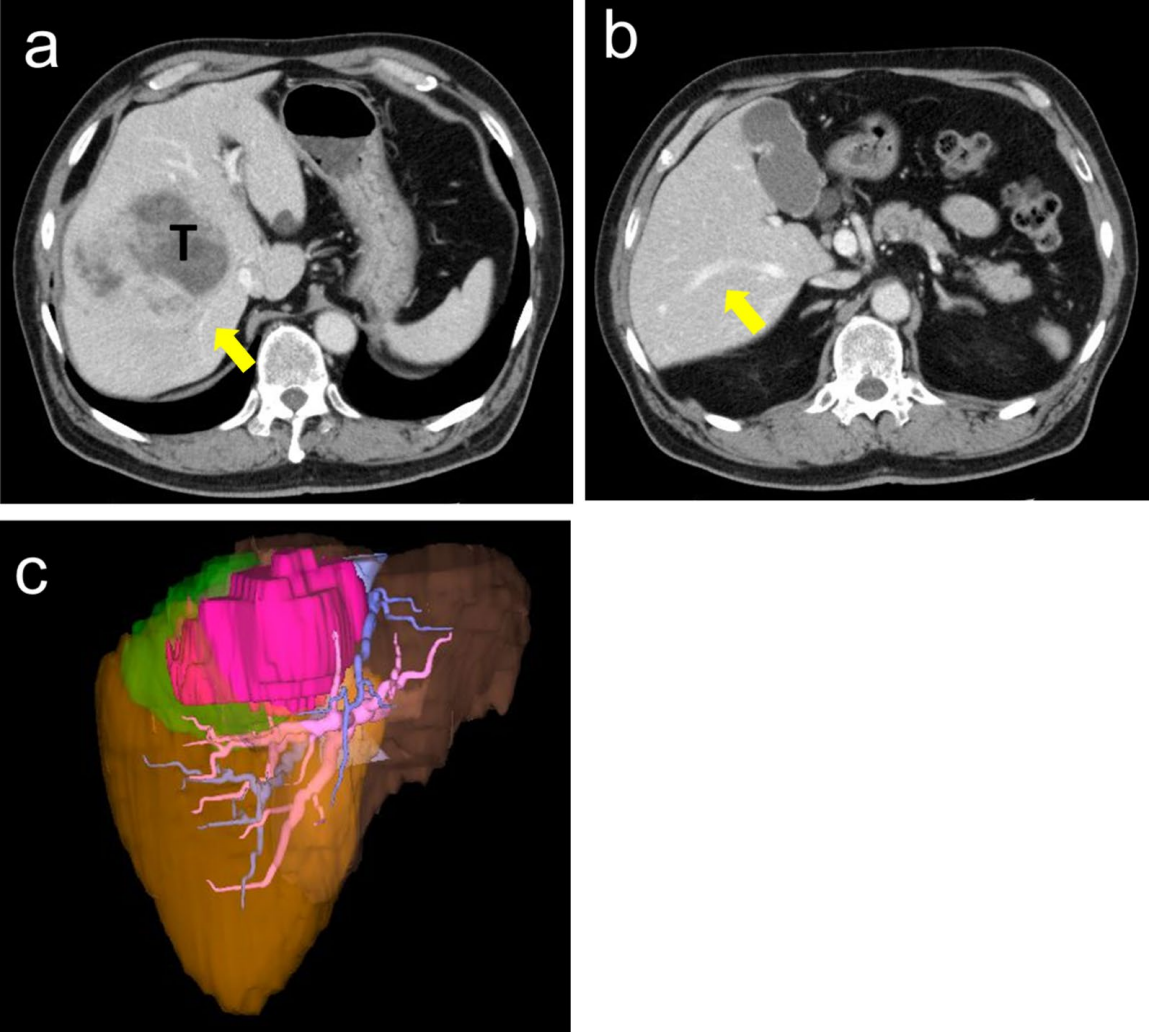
Hepatocellular carcinoma occupying segments 7 and 8 involved the RHV. a Computed tomography showed the tumor located in segments 7 and 8. The tumor involved the RHV (yellow allow). b The thick IRHV (yellow arrow) was observed. c 3D CT imaging of the RHV (green), IRHV (orange) drainage areas, and tumor (pink). RHV; right hepatic vein. IRHV; inferior right hepatic vein. 3D CT, three-dimensional computed tomography
